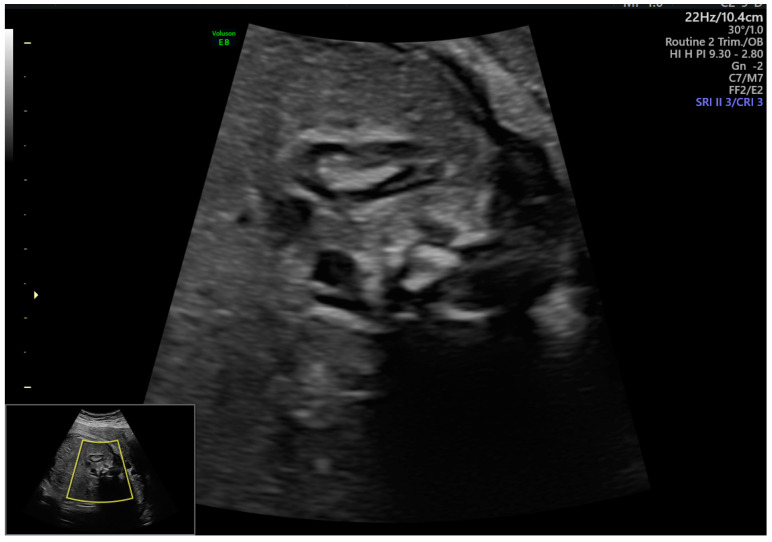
Sonographic assessment of fetal adrenal gland morphology.
